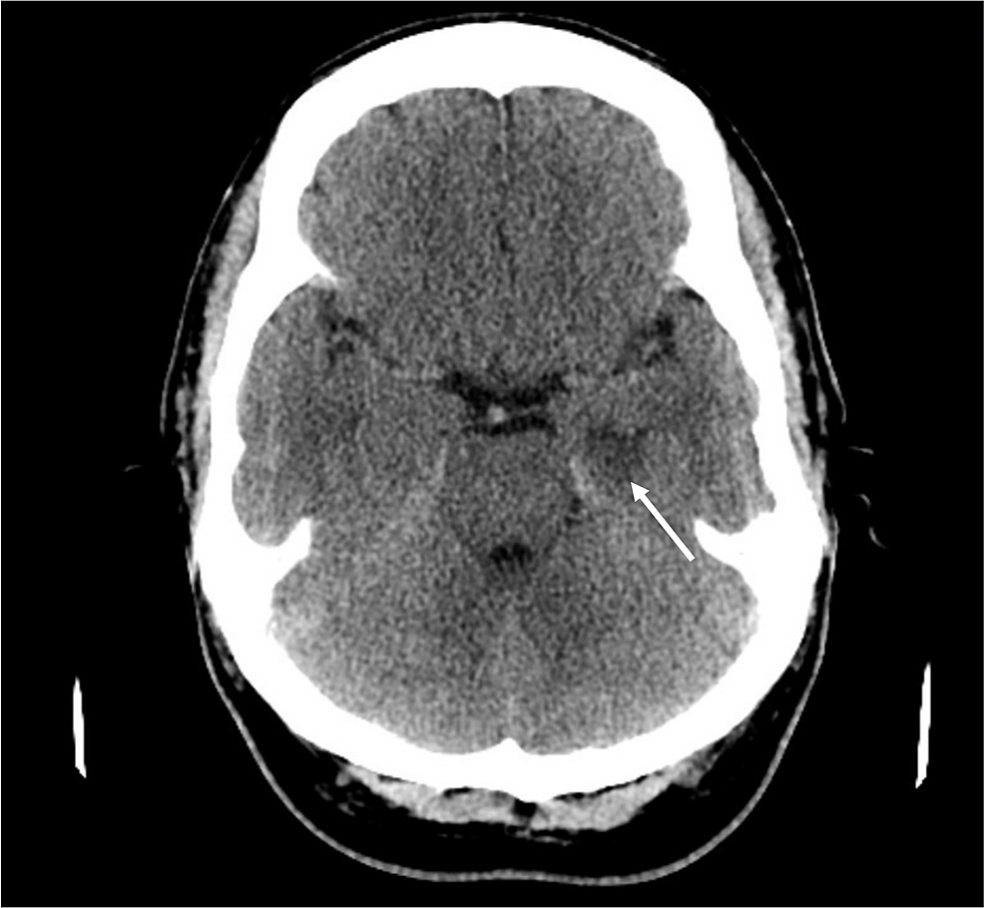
Axial computed tomography (CT) of the brain showing asymmetric hypoattenuation at the left medial temporal lobe indicating edema (white arrow)
